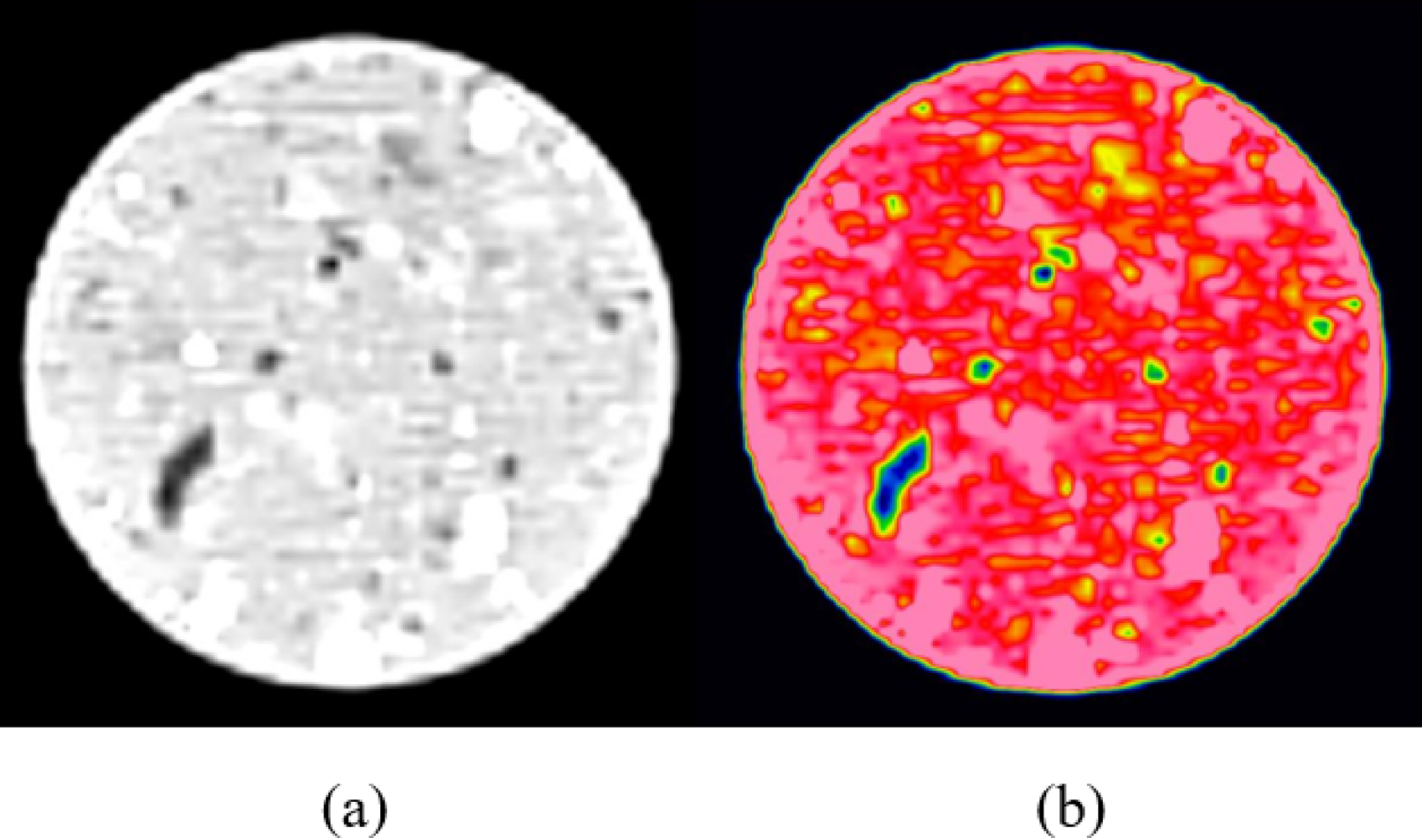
Pseudocoloring of CT Scan Images. (a) before pseudocoloring, (b) after pseudocoloring.
